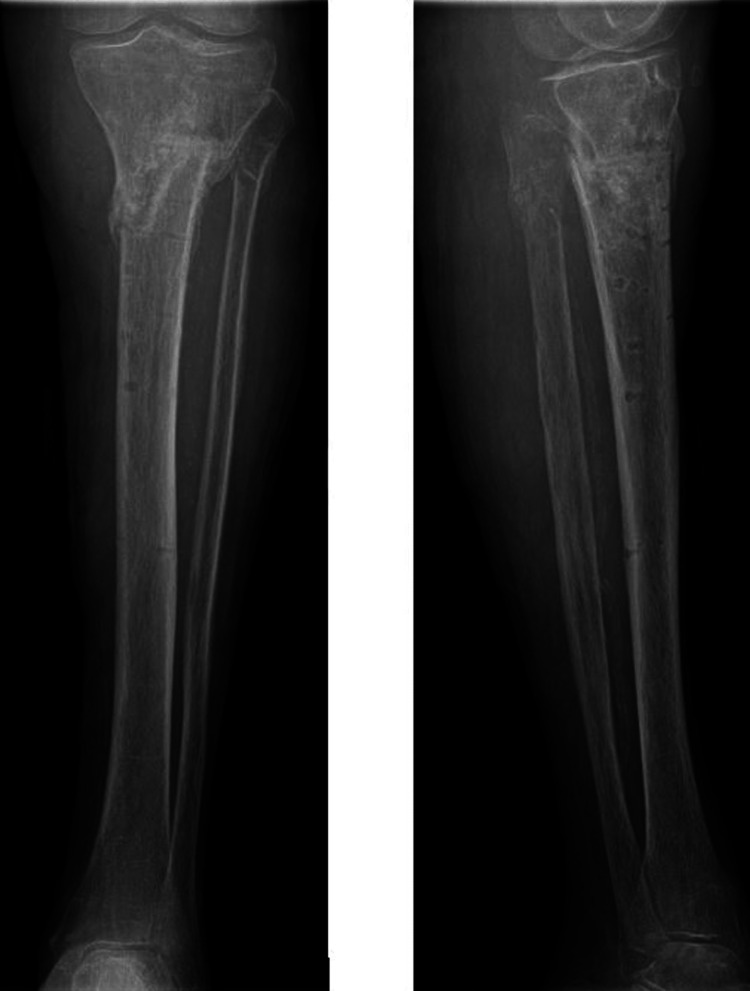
Anteroposterior and lateral X-rays of the left tibia.
